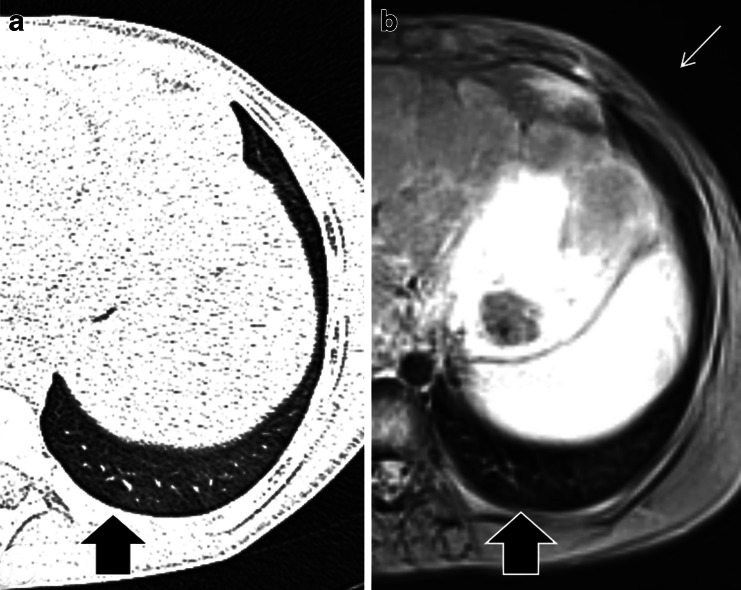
Radial k space-filling (PROPELLER) artifact and trapped air assessment. a CT versus (b) free-breathing spin echo MRI with radial k space trajectory in a 24-year-old woman with cystic fibrosis. End-expiration scans performed on the same day. Note streak artifacts outside the chest (*thin arrow*) due to the helicoidal k-space reconstruction. Note also area of trapped air in the left lower lobe (*thick arrow*s)
